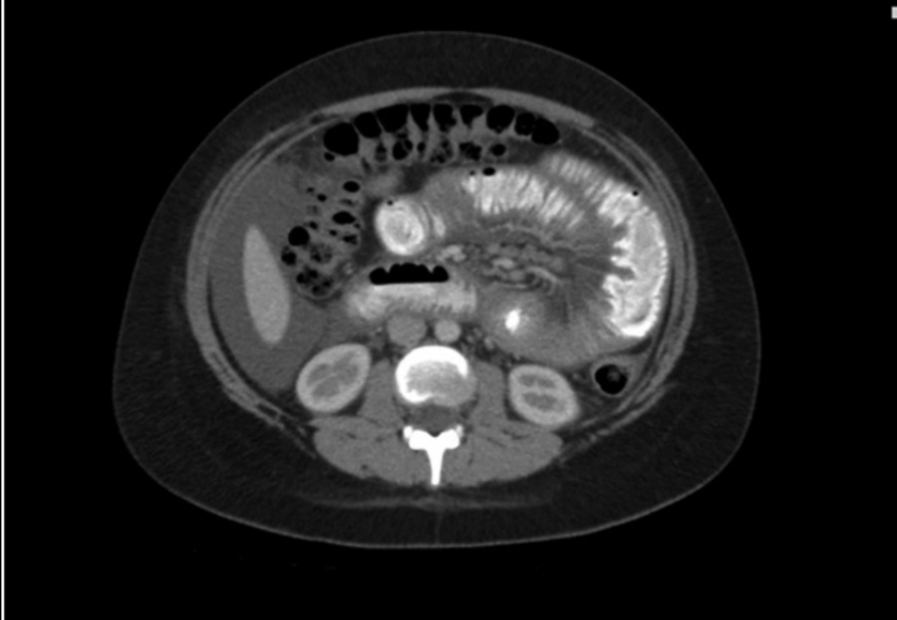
CT scan of the abdomen Diffuse bowel wall thickening with extensive abdominal and pelvic ascites.
